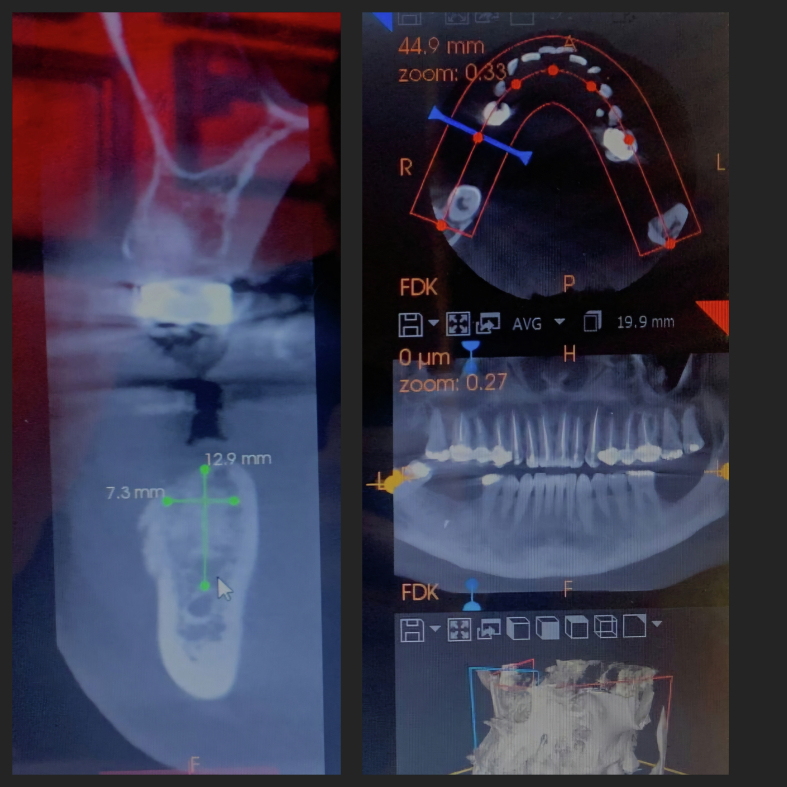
CBCT showing bone gain in vertical and horizontal dimensions in first molar area.
